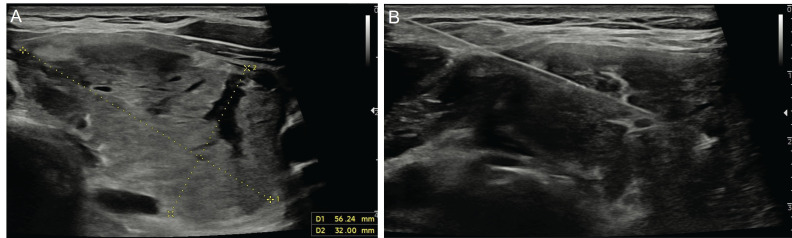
Ultrasonography image of a 35-year-old male patient shows a 56x32-mm nodule (A) located in the left lobe of the thyroid gland after three negative fine-needle aspiration biopsy results. The patient underwent a core needle biopsy with a 20G-10cm co-axial semi-automatic biopsy system (Geotek, Turkiye) (B) and was diagnosed with follicular neoplasia.
